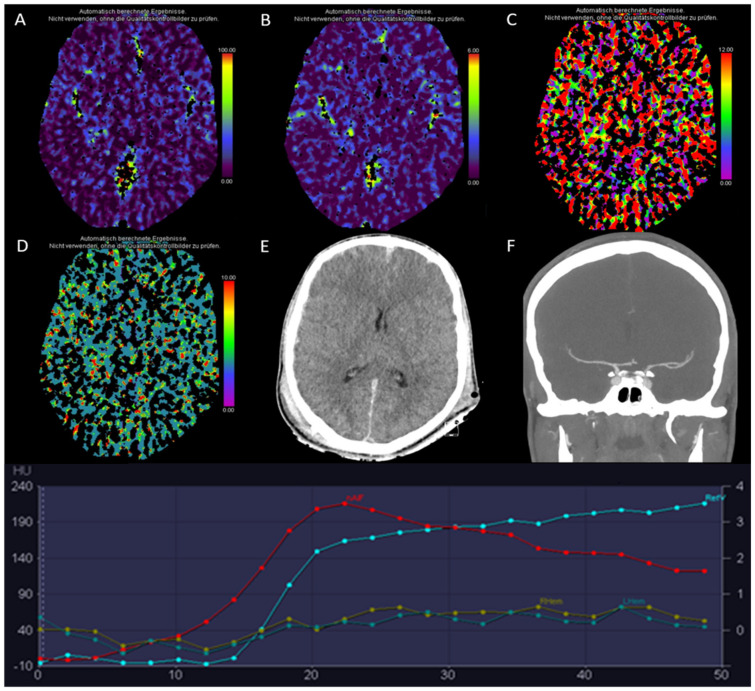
Color-coded maps generated from computed tomography perfusion (CTP) of the brain: (A) cerebral blood flow (CBF) [mL/100 g/min], (B) cerebral blood volume (CBV) [mL/100 g], (C) time to maximum (Tmax) [s], and (D) mean transit time (MTT) [s]. (E) Non-enhanced CT (NECT) and (F) arterial CT angiography (CTA). This patient was referred to the hospital after a traffic accident with a Glasgow Coma Scale (GCS) of 3. Besides skull fractures and a subarachnoid hemorrhage, NECT showed general brain swelling, loss of the grey to white matter differentiation, and severe reduction in the cerebrospinal fluid spaces due to a general cerebral edema. There was no detectable CBF or CBV according to CTP. On the CTA, the contrast in the intracranial arteries was still visible, but hardly recognizable in the veins. The time attenuation curve of the CTP showed that this was not the result of a technical failure or methodological issues: there was a regular increase in the arterial curve (red line) but a plateau in the venous curve (blue line), most likely due to the lack of parenchymal brain perfusion (right hemisphere: bright green line; left hemisphere: dark green line).
